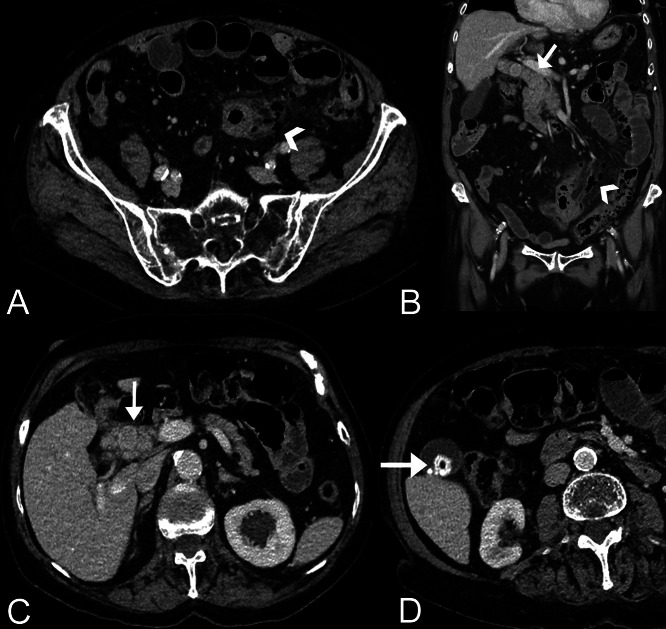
(A-D) (A and B) Contrast-enhanced CT in transversal and coronal reconstruction reveals Meckel diverticulitis (white arrowhead) situated in the distal jejunum (left abdomen), accompanied by local peritonitis and numerous surrounding extraluminal gas bubbles indicative of perforation. Additional findings include signs of subileus. (C and D) Cholelithiasis is also depicted, with marked choledocholithiasis (white arrows).
